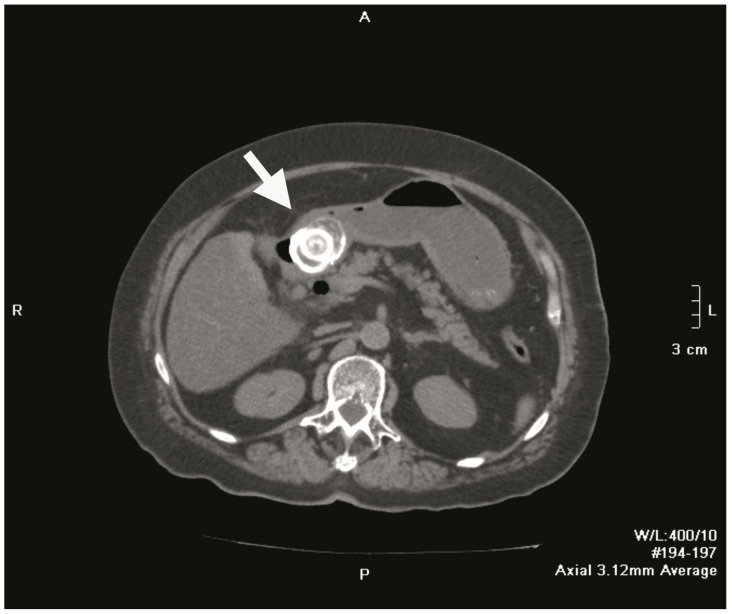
Axial non-contrast CT image of the upper abdomen revealing a large gallstone (white arrow) lodged in the duodenal bulb, a finding characteristic of Bouveret’s syndrome; the gallstone is obstructing gastric outflow and causing marked gastric distention. An air-fluid level is observed within the distended stomach.
